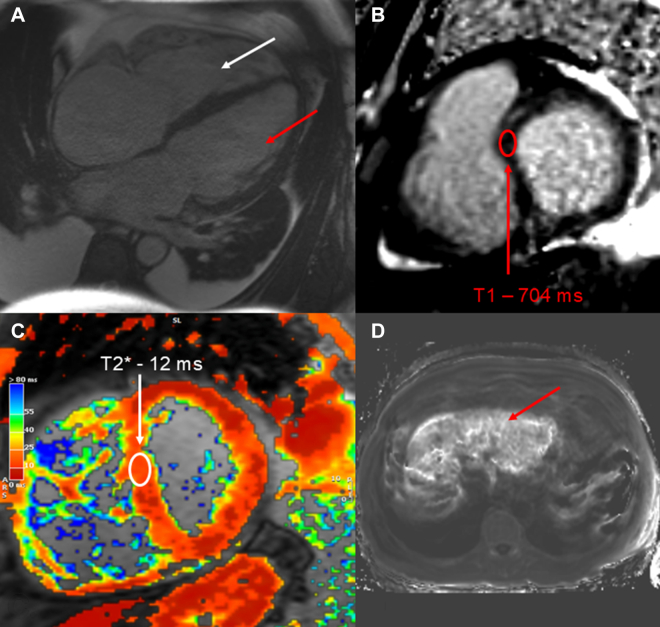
Cardiac and Liver Magnetic Resonance Imaging Findings of Patient Case 1 (A) Four-chamber view on cardiac magnetic resonance imaging (T1 weighted) showing severe biventricular (white arrow points to the right ventricle and red arrow to the left ventricle) and biatrial enlargement. (B) Short-axis cardiac magnetic resonance imaging T1 map at the mid-level, with the arrow pointing to the low septal T1 value of 704 ms. (C) T2∗ mapping. Red shading in the left ventricle represents a short T2∗ relaxation time. The white arrow points to the measured septal T2∗ relaxation time of 12 ms, consistent with iron overload. (D) Liver magnetic resonance imaging R2∗ sequence showing hyperintensity in the liver (red arrow) compared with other abdominal viscera, suggesting hepatic iron overload.
